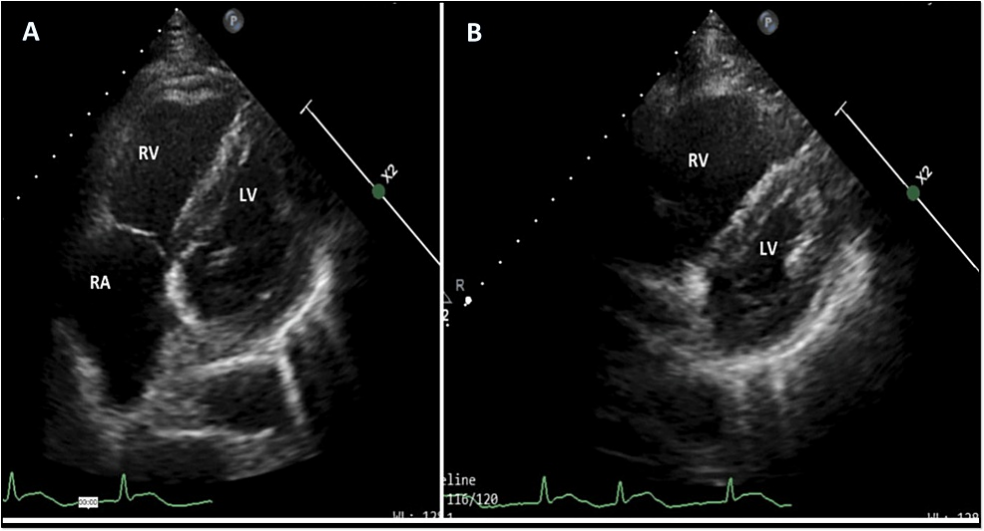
Transthoracic echocardiography Echocardiography showing RA, RV dilatation (A), and D-shaped septum (B) RA: right atrium, RV: right ventricle
